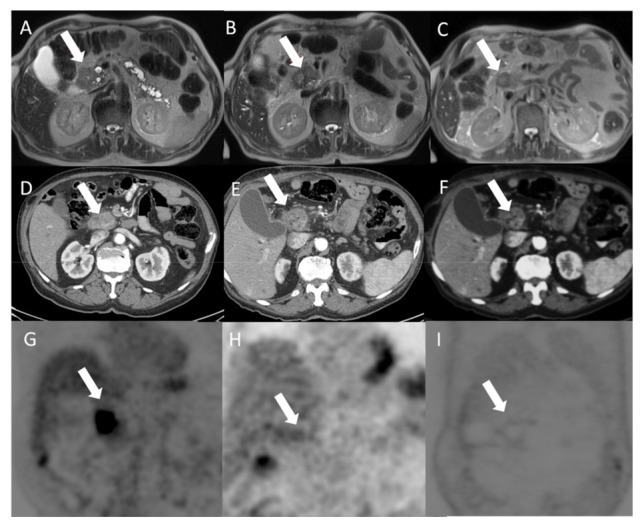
Patient with adenocarcinoma of the pancreatic head treated with variable geometry. Before treatment, the arrow identifies the lesion on the HASTE T2-W sequence (A); after one month of treatment, on the HASTE T2-W sequence (B), there were not significant differences in signal intensity and lesion size; after six months from ECT, the HASTE T2-W sequence (C) showed significant differences in signal intensity and lesion size. In the pancreatic phase of the CT study (D), the lesion appeared hypodense (arrow). After one month of treatment, the lesion appeared more hypodense (E); after six months of treatment, on CT (F), there was also a significant reduction of the lesion size. Also, PET evaluation between pre- (G) and post-treatment detected a reduction of glucose uptake both after 1 month (H) and after 6 months (I) by ECT. According to data, the patient, after one month and after six months, was classified to be in partial response by ECT.
